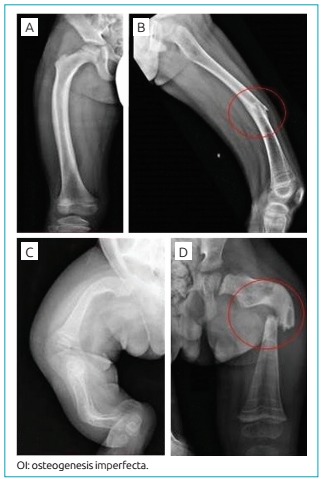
Radiographic findings of the lower limbs. (A and B) Male patient with osteogenesis imperfecta type 1 showing minor bowing of the right femur and diaphyseal fracture of the left femur; (C) female patient with osteogenesis imperfecta type 3 showing severe bowing of the long bones; (D) male patient with osteogenesis imperfecta type 3 showing significant diaphyseal fracture and osteoporosis.
